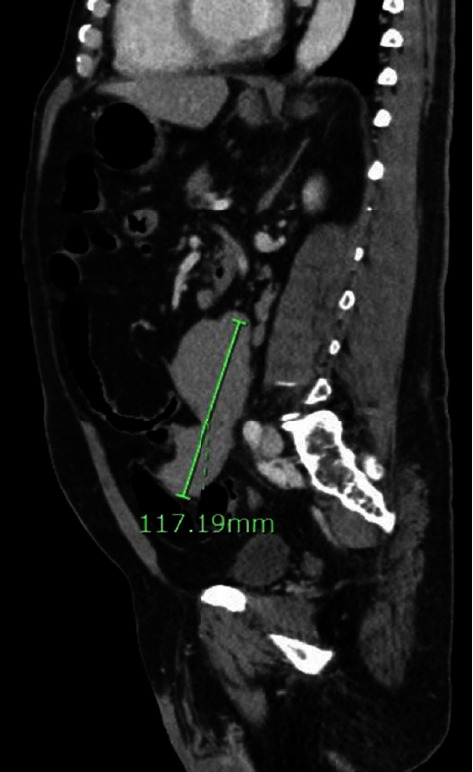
The same mass that was visualized in Figure 3, measured as 11.7 cm on sagittal view of CT.
